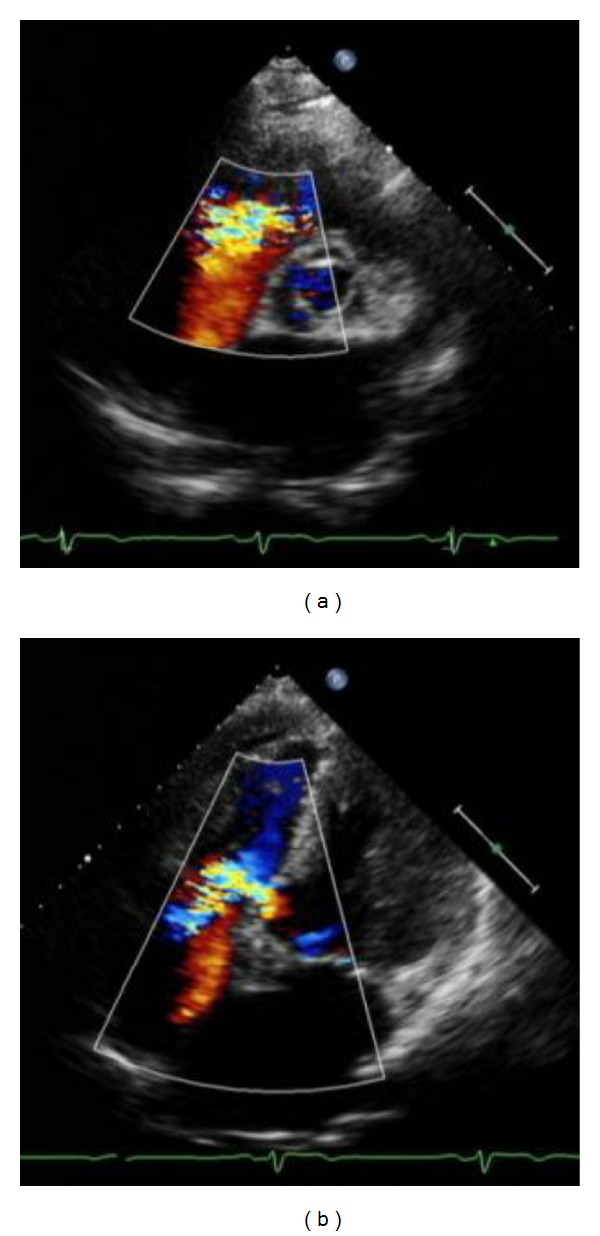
Echocardiography: short axis (a) and apical 4-chamber (b) view. Perimembranous ventricular septal defect *L* → *R* flow and moderate tricuspid regurgitation flow were seen.
